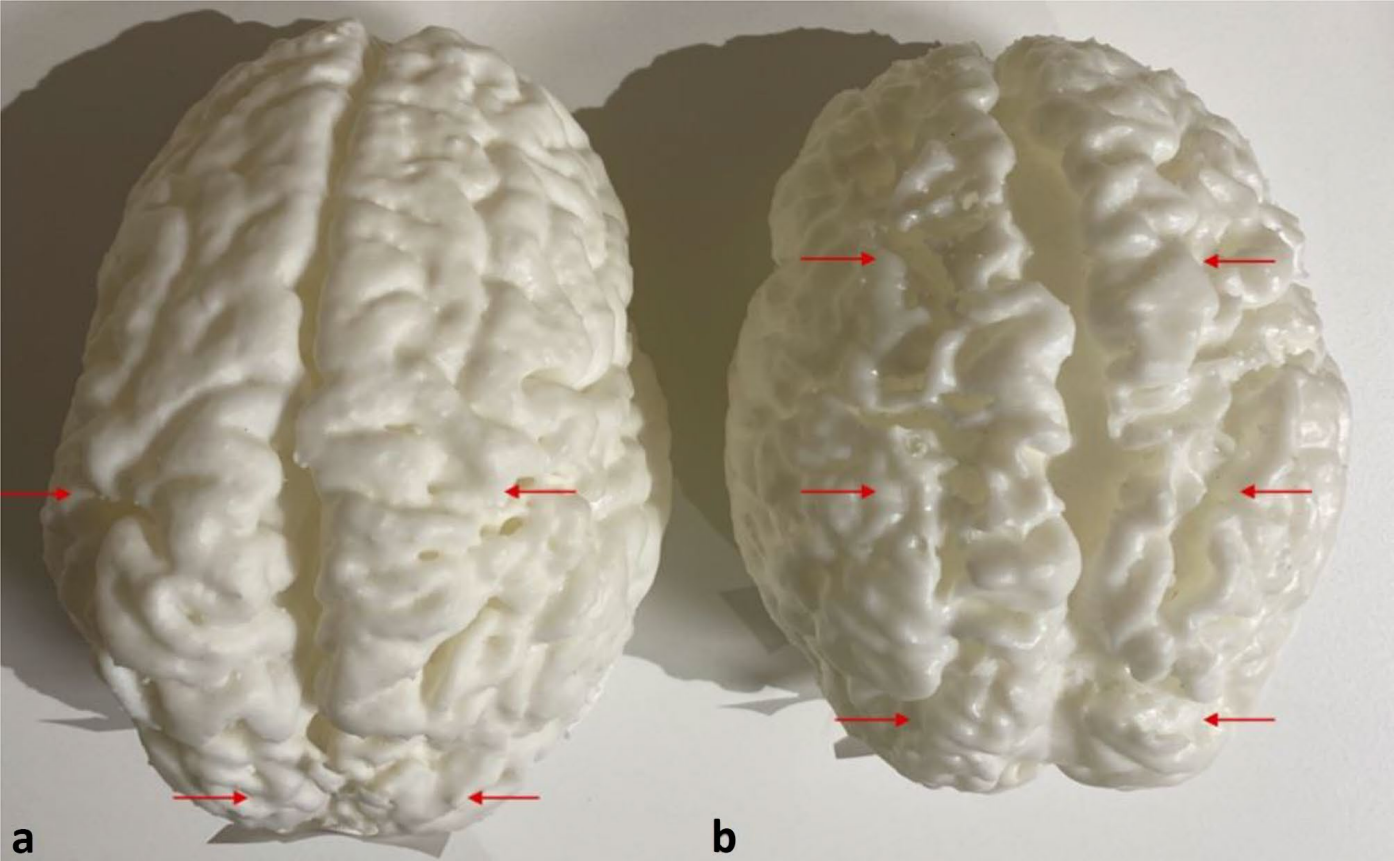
Two models of different children’s brains side by side for comparison. Both patients suffered partial prolonged hypoxic ischemic injury at term gestation, now with cerebral palsy, who had delayed MR imaging — a 2-year 2-month-old boy (a) and a 11-year 6-month-old boy (b). Note, the more severe atrophy in the 11-year-old (b) evident from increased depth and width of the sulci in the watershed region (red arrows in each model) as well as the low overall brain volume (i.e., an 11-year-old with a similar or smaller brain volume than a 2-year-old) when visualizing the models side by side
